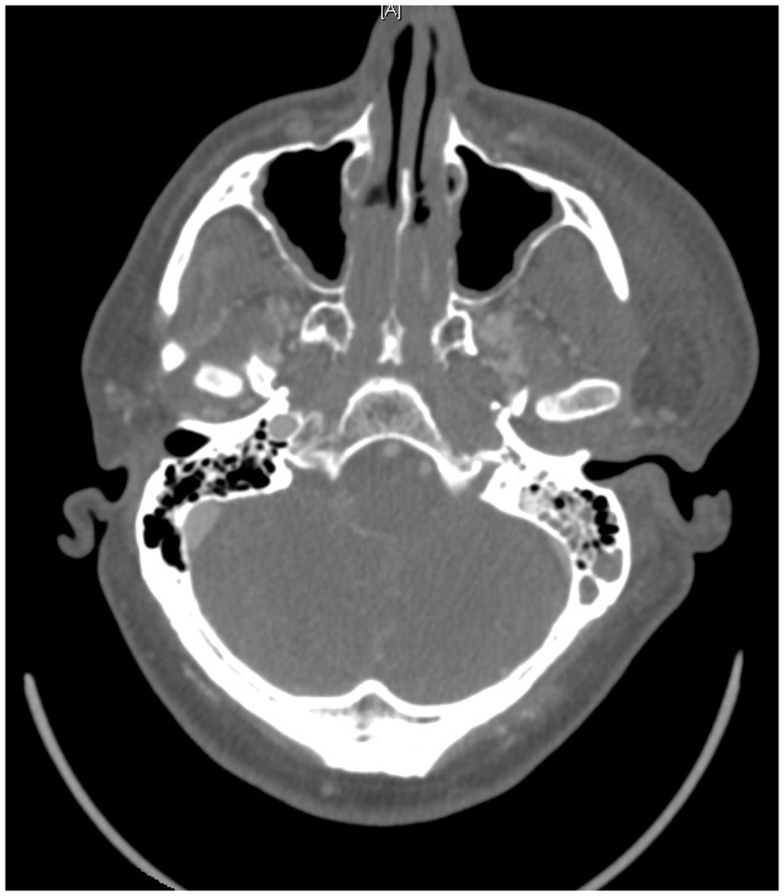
Post-operative follow up CT scan showing lack of flow through the left ICA.
